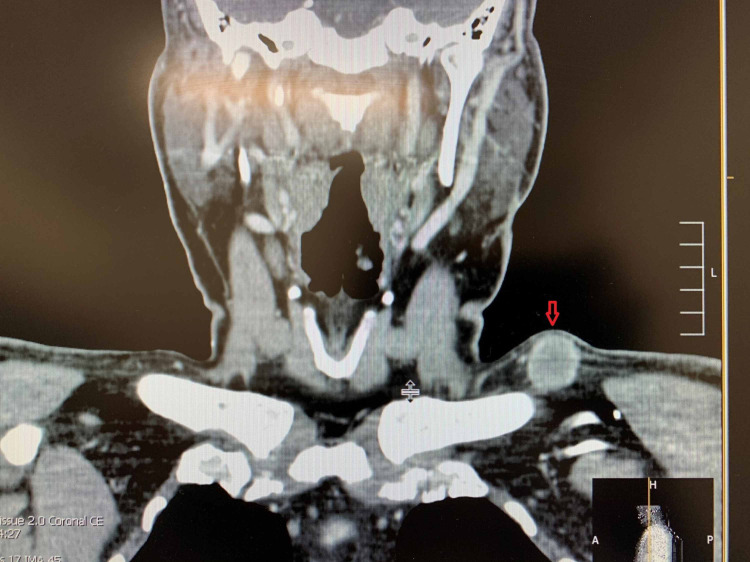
CT neck Red downward arrow: enlarged left supraclavicular lymph node CT: computed tomography
